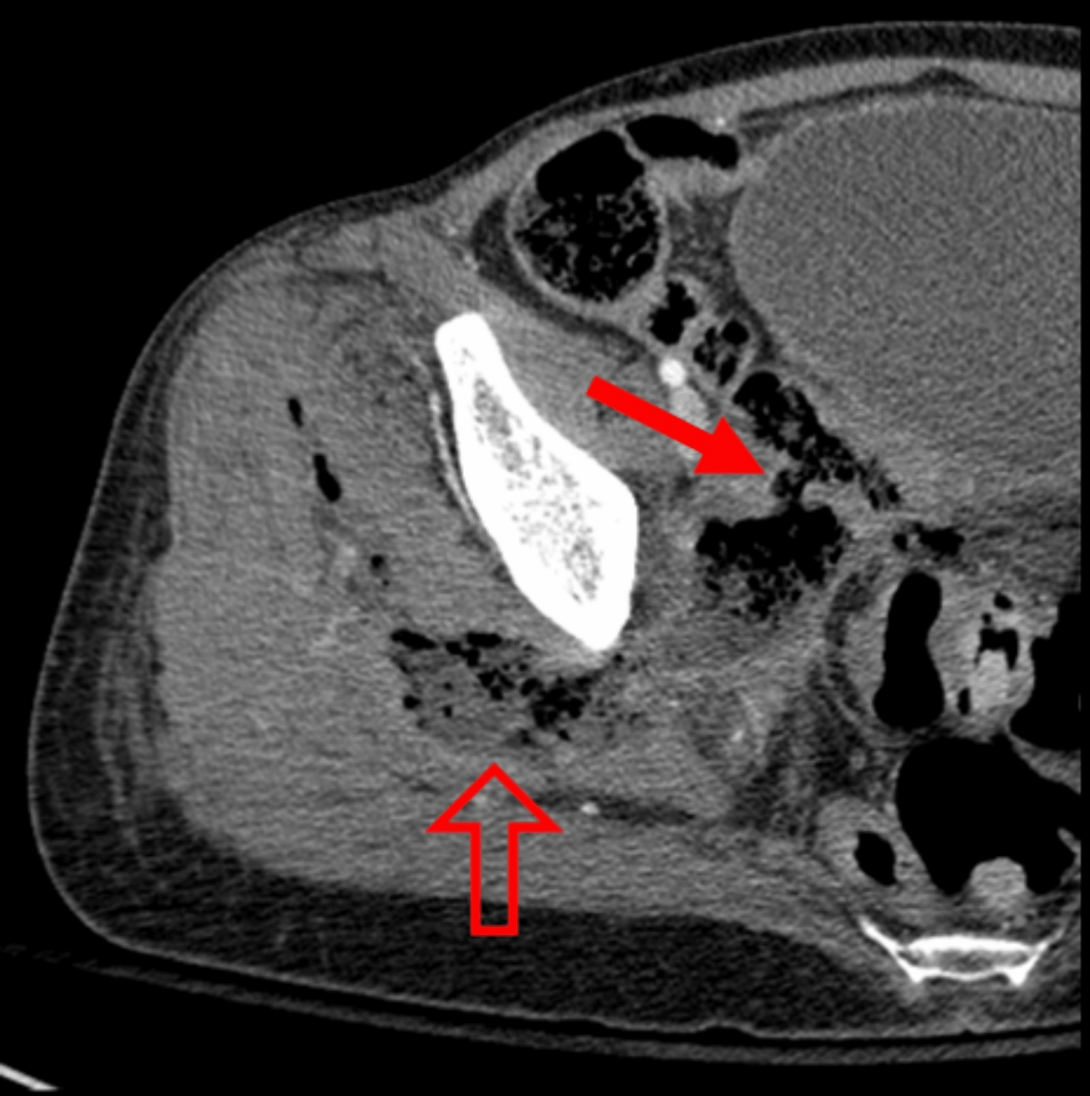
Fistula tract extending from a distal small bowel loop to an intrapelvic fluid collection. The patient underwent CT imaging of the abdomen and pelvis with intravenous (IV) contrast, which demonstrated a complex collection of fluid and gas in the right hemipelvis, extending into the iliopsoas muscle and gluteal musculature (unfilled arrow). A thin tract is visible along the anterior aspect of the intrapelvic fluid collection, extending to and appearing contiguous with a distal small bowel loop located posteromedial to the cecum (arrow).
